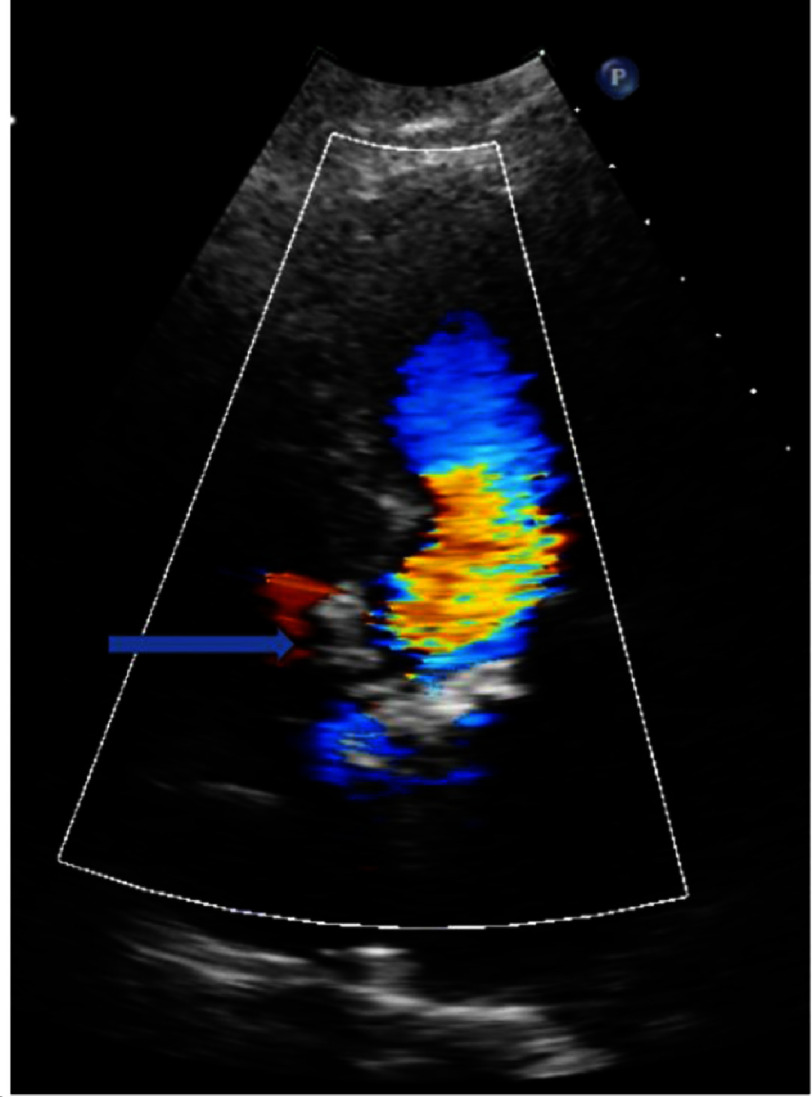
Transthoracic echocardiography demonstrating membranous ventricular septal defect.
